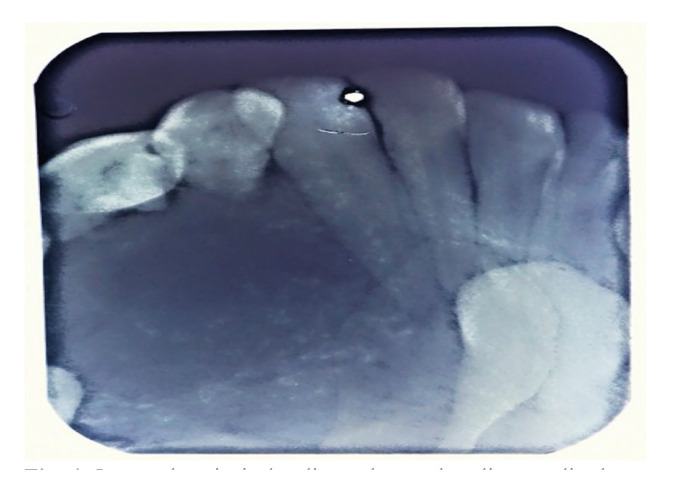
Intraoral periapical radiograph reveals solitary radio-dense mass with internal calcifications enclosing the impacted 34, mesially displacing the roots of 31 and 32.
